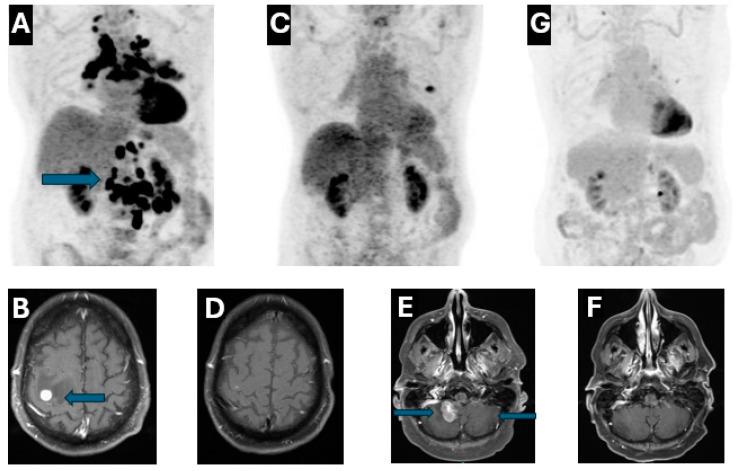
(A), *PET-CT*: Baseline tumor burden including duodenal metastasis (12/2019); (B), *MRI brain:* Brain metastasis at the diagnosis (12/2019); (C), *PET-CT*: 3 months after chemoimmunotherapy (4/2020); (D), *MRI brain*: 3 months after chemoimmunotherapy (4/2020); (E), *MRI brain*: Baseline with cerebellar metastasis and LMD (6/2022); (F), *MRI brain*: 6 weeks after chemotherapy and tepotinib (7/2022); (G), *PET-CT*: 15 months after re-starting tepotinib (6/2024).
